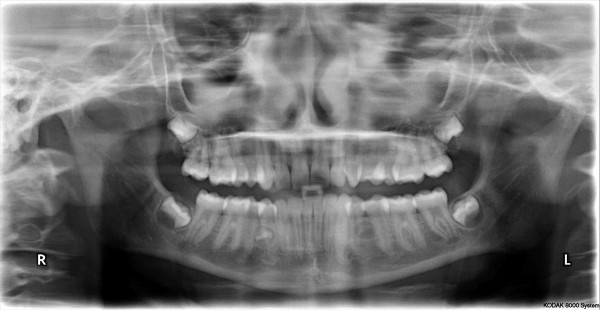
Orthopantomography. Image shows a bilateral opacity of the maxillary sinuses.
